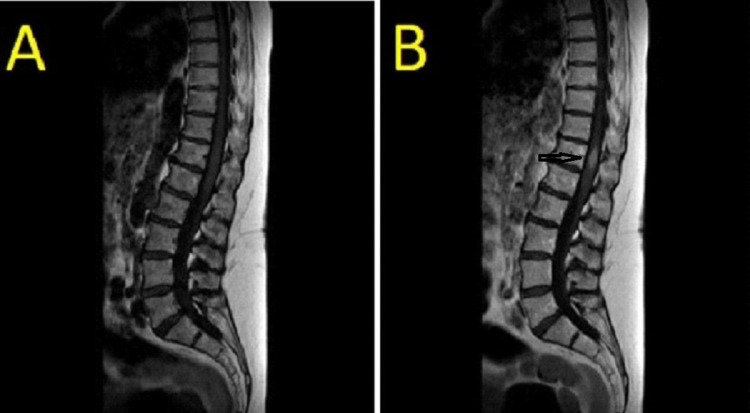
MRI non-contrast (A), MRI with gadolinium contrast showing “flame sign” (B)
